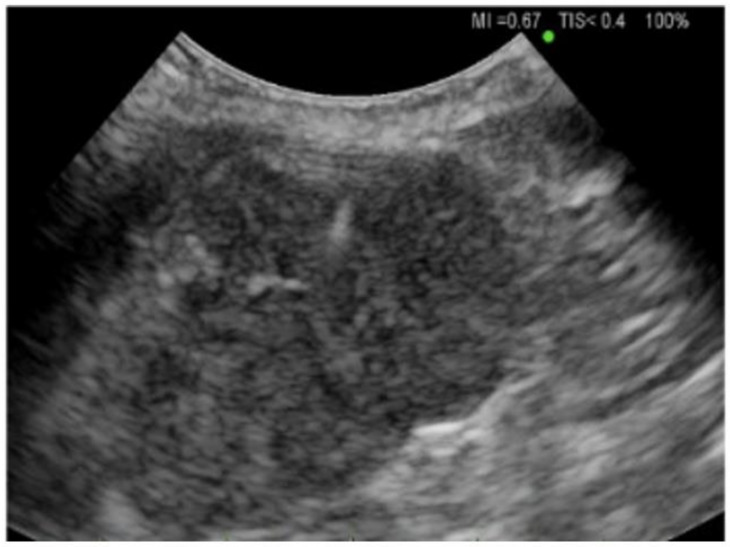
Ultrasound of a Supraclavicular Lymph Node. The lymph node is lobular, non-calcified and irregular which was consistent with metastatic lung cancer.
